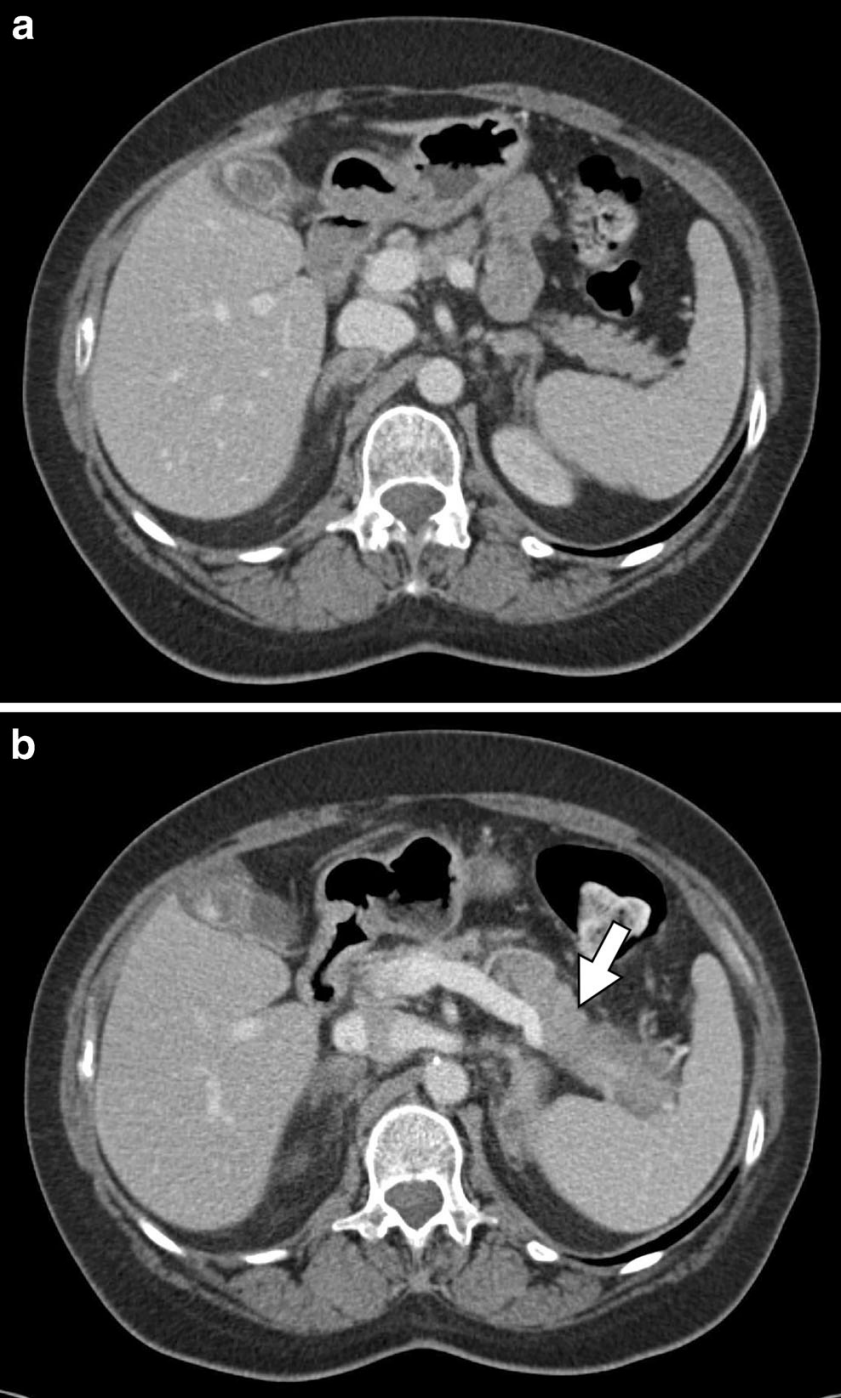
A 59-year-old female patient with stage IV non-small cell lung carcinoma (NSCLC) and known metastatic disease to the adrenal glands treated with carboplatin, premetrexed, and bevacizumab. a Axial contrast-enhanced CT shows a normal pancreas prior to chemotherapy. b Post-treatment axial contrast-enhanced CT demonstrating diffuse oedema and parenchymal enlargement (*arrow*) consistent with the development of acute interstitial pancreatitis
